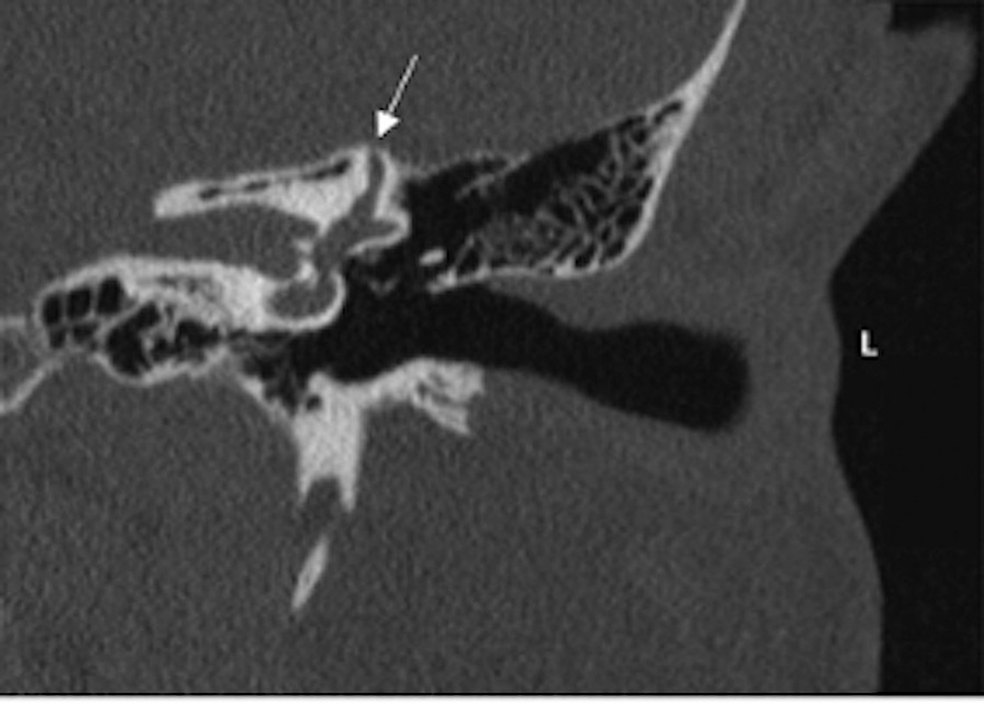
A 37-year-old woman presenting with hyperacusis, tinnitus, and vertigo. High-resolution CT scan of her left temporal bone shows her (L) left superior semicircular canal. The white arrow indicates thinning of arcuate eminence. Citation from Preet et al. [11] CT, computed tomography
